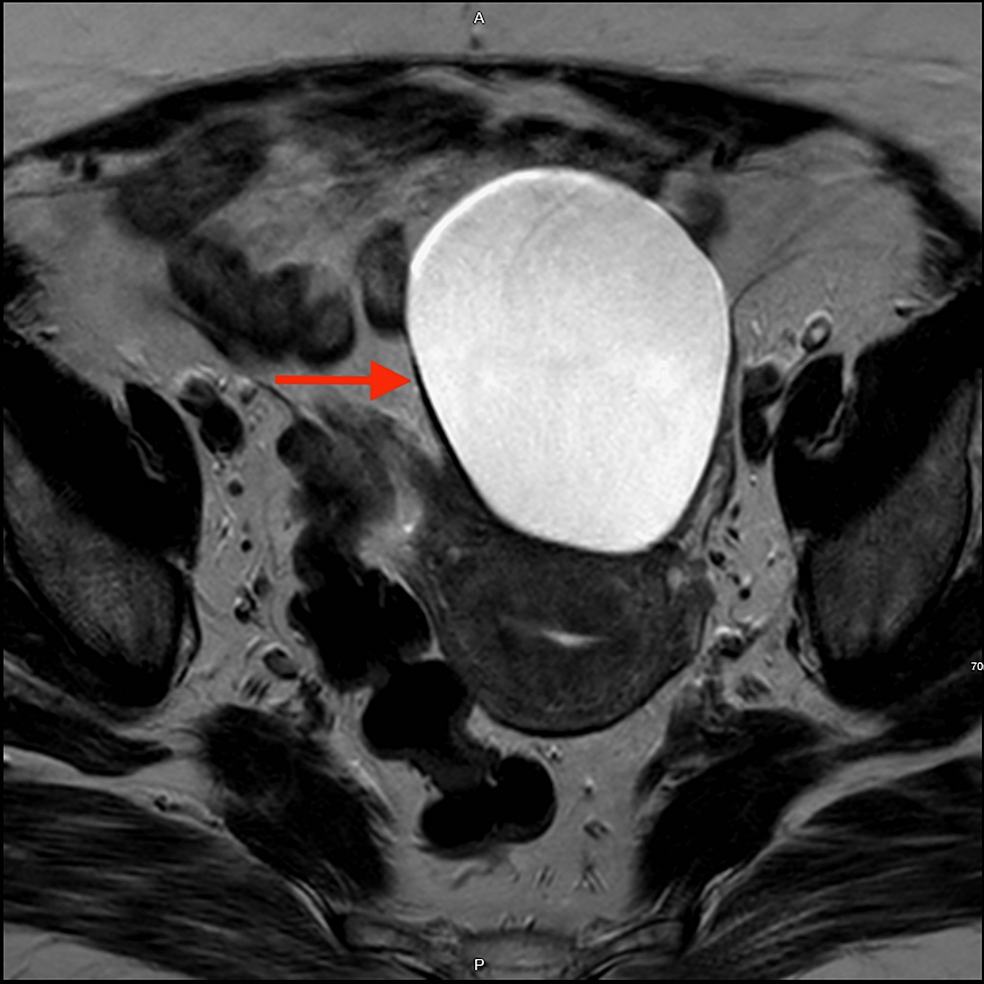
Magnetic resonance imaging (MRI) axial view. Left adnexal unilocular cyst mostly arising from the left ovary. It shows a thin-walled with no soft tissue component, papillary projection, or internal septations. T1 and T2 showed low and markedly high signal intensity, respectively, without diffusion restriction or enhancement.
